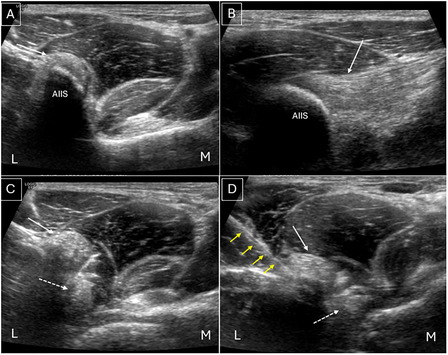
Rectus femoris peritendinous injection. Transverse (A) and longitudinal (B) ultrasound images of the origin of the direct head (solid arrow) in short and long axis, respectively. Slightly distal with the transducer in a transverse orientation, the direct (solid arrow) and indirect (dashed arrow) heads are visible in short axis (C). During injection at the level of figure part (C), the needle (yellow arrows) is advanced in plane to the transducer and positioned deep to both heads (white arrows) where the therapeutic steroid and anesthetic mixture is injected (D). (AIIS, anteroinferior iliac spine; L, lateral; M, medial.)
